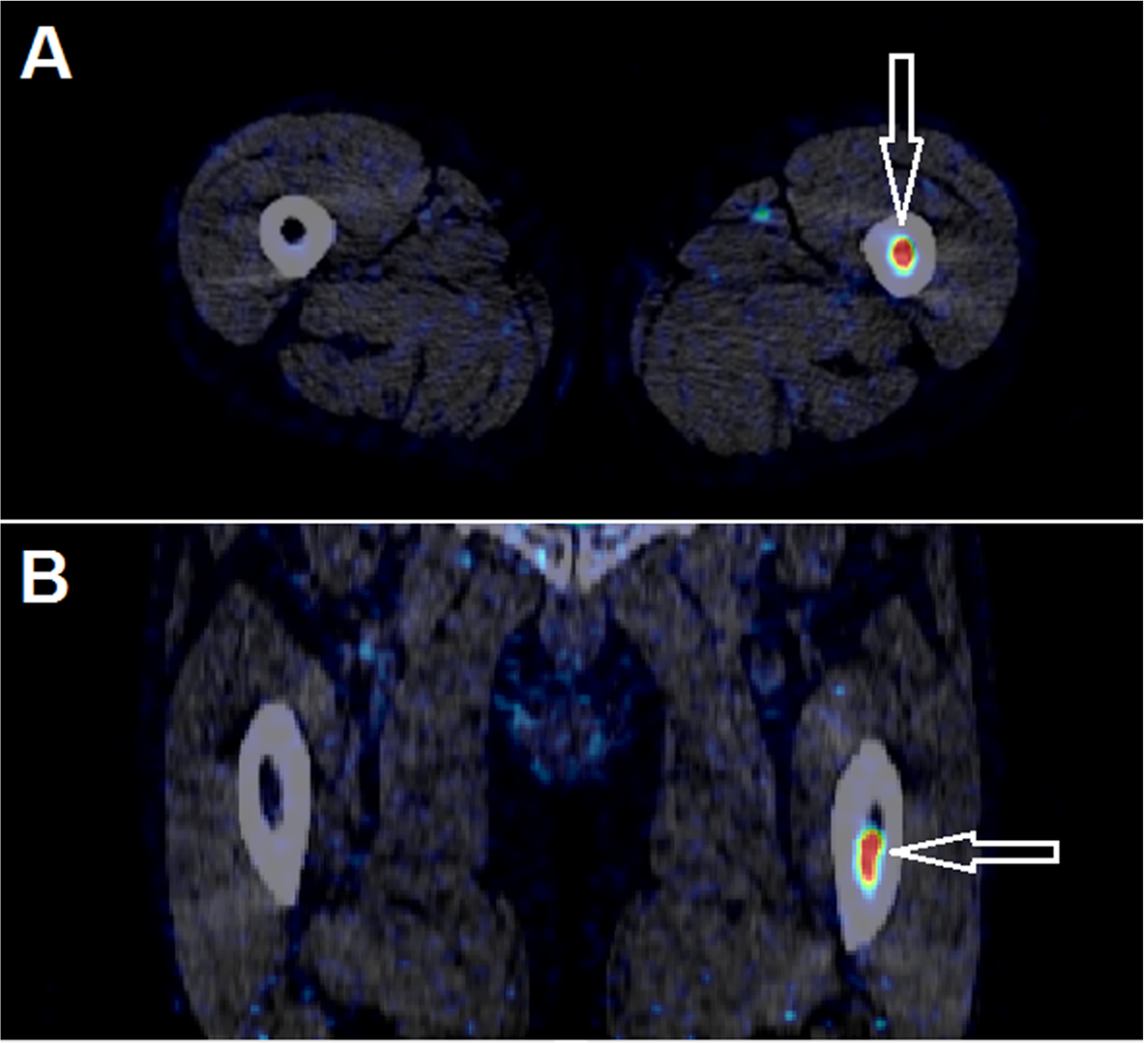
Arrows* show a metastasis in the left femoral bone of patient #6 detected on a static ^18^F-FDGal PET image (visible on both static images; here, the 1-h static image is shown). a Transaxial slice. b Coronal slice
